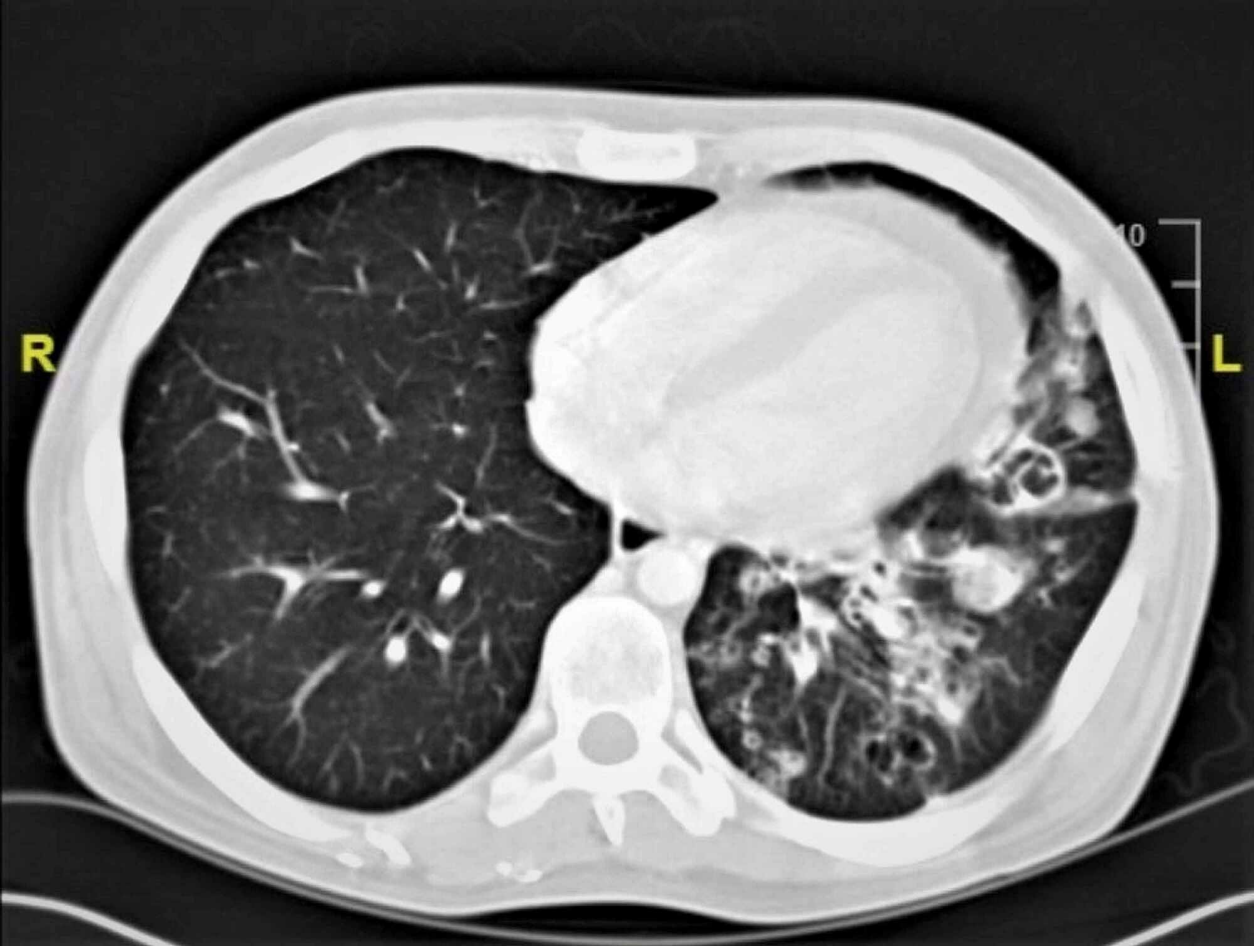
CT chest CT chest shows improvement of the pulmonary consolidation with residual cavitation consistent with a necrotizing process.
